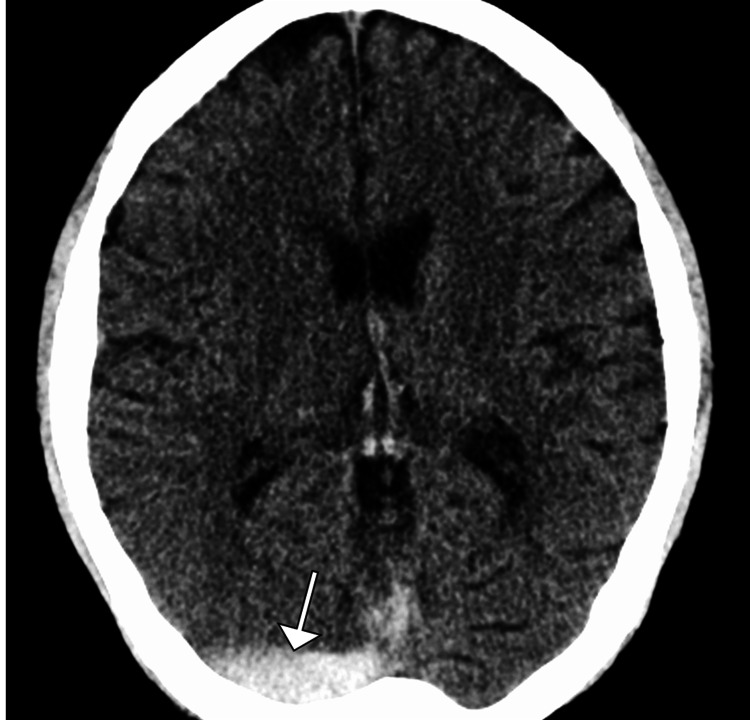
Axial non-contrast CT head shows hyperdensity along the right straight and transverse sinus (arrow) CT: computed tomography
